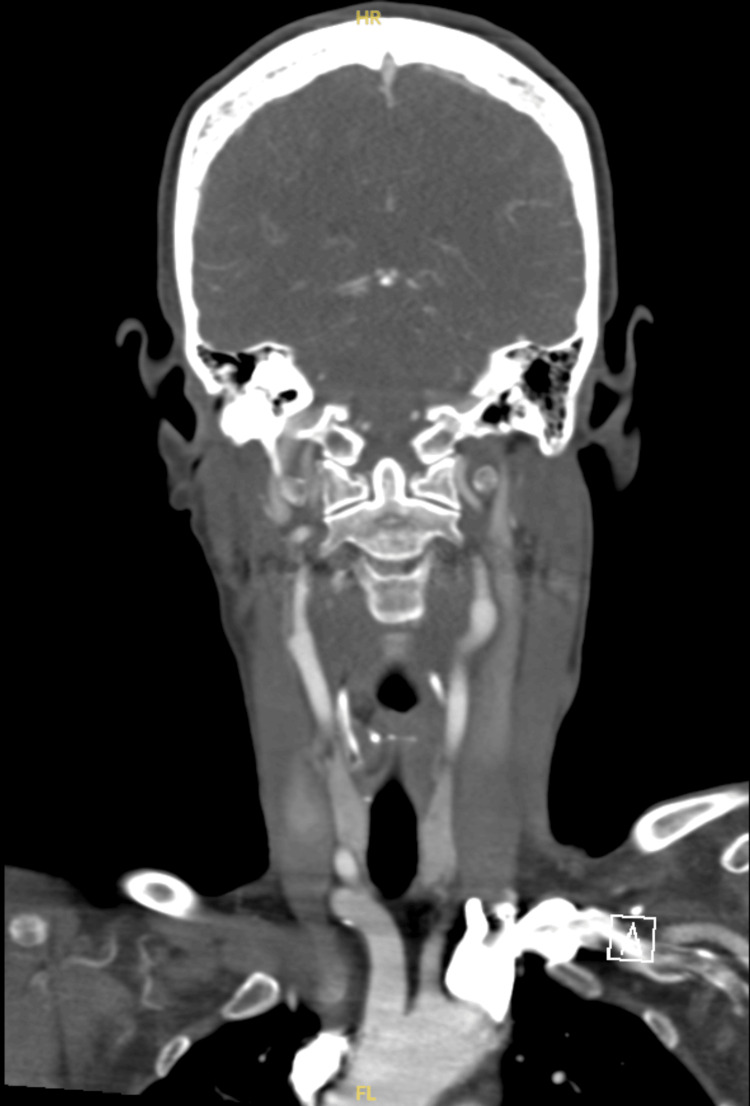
CT aortic arch showing no obvious abnormality
